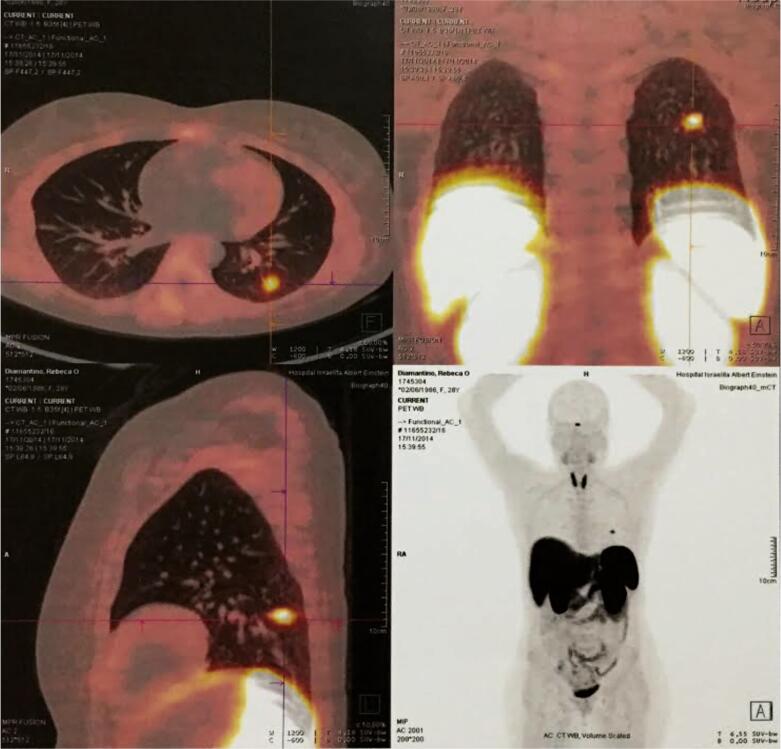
68Ga-DOTA-TATE PET-CT showing anomalous tracer uptake: a regular, solid, non-calcified lung nodule, located between the superior and basal posterior segments of the left lung inferior lobe that measured 1.3 x 0.9 cm in diameter, with an SUV of 6.1, consistent with a tumor of neuroendocrine origin (carcinoid tumor).
